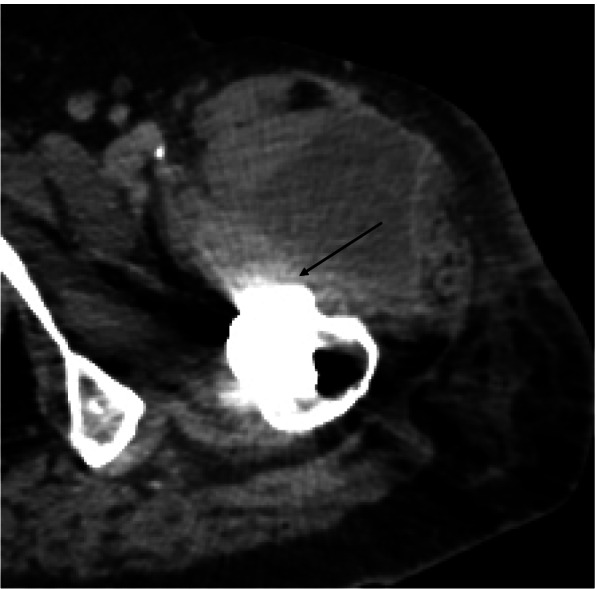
Axial CT shows field accumulation anterior to femoral bone. Due to beam hardening (black arrow), the soft tissue surrounding the periprosthetic bone is not evaluable
